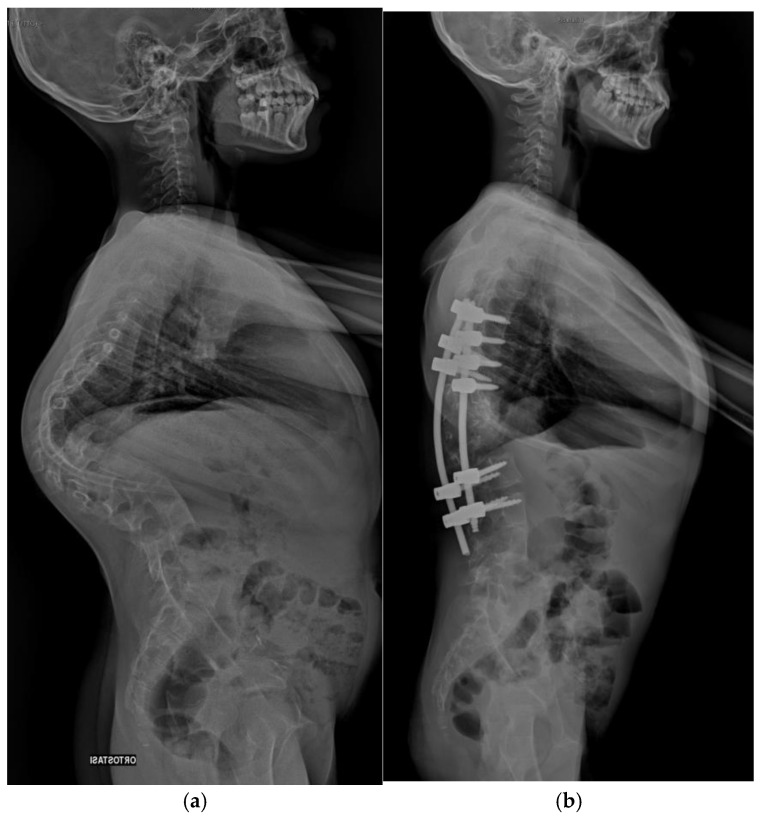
Lateral radiograph of Patient 2. (a) Preoperative assessment. (b) Postoperative assessment.
